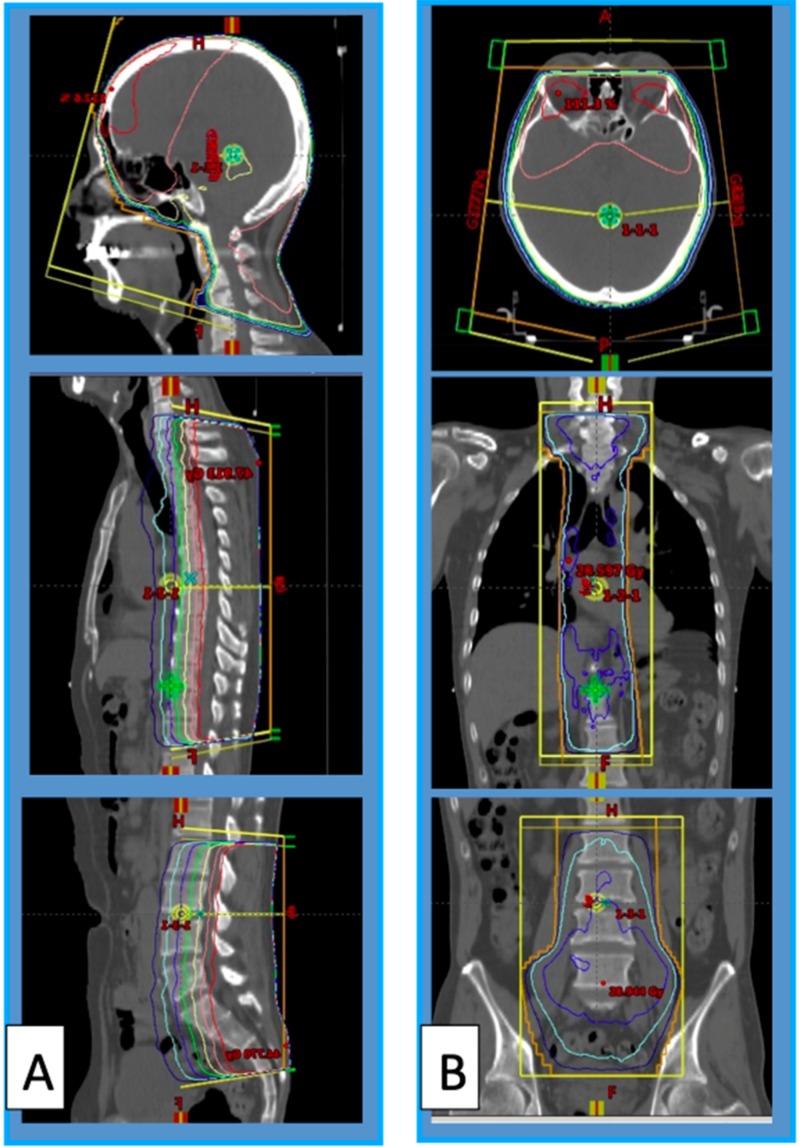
Dose planning of craniospinal irradiation Lateral views (A), axial (B, upper), and frontal views (B, middle and lower). High-uptake areas of fluorodeoxyglucose (FDG) were covered by 95% dose line.
